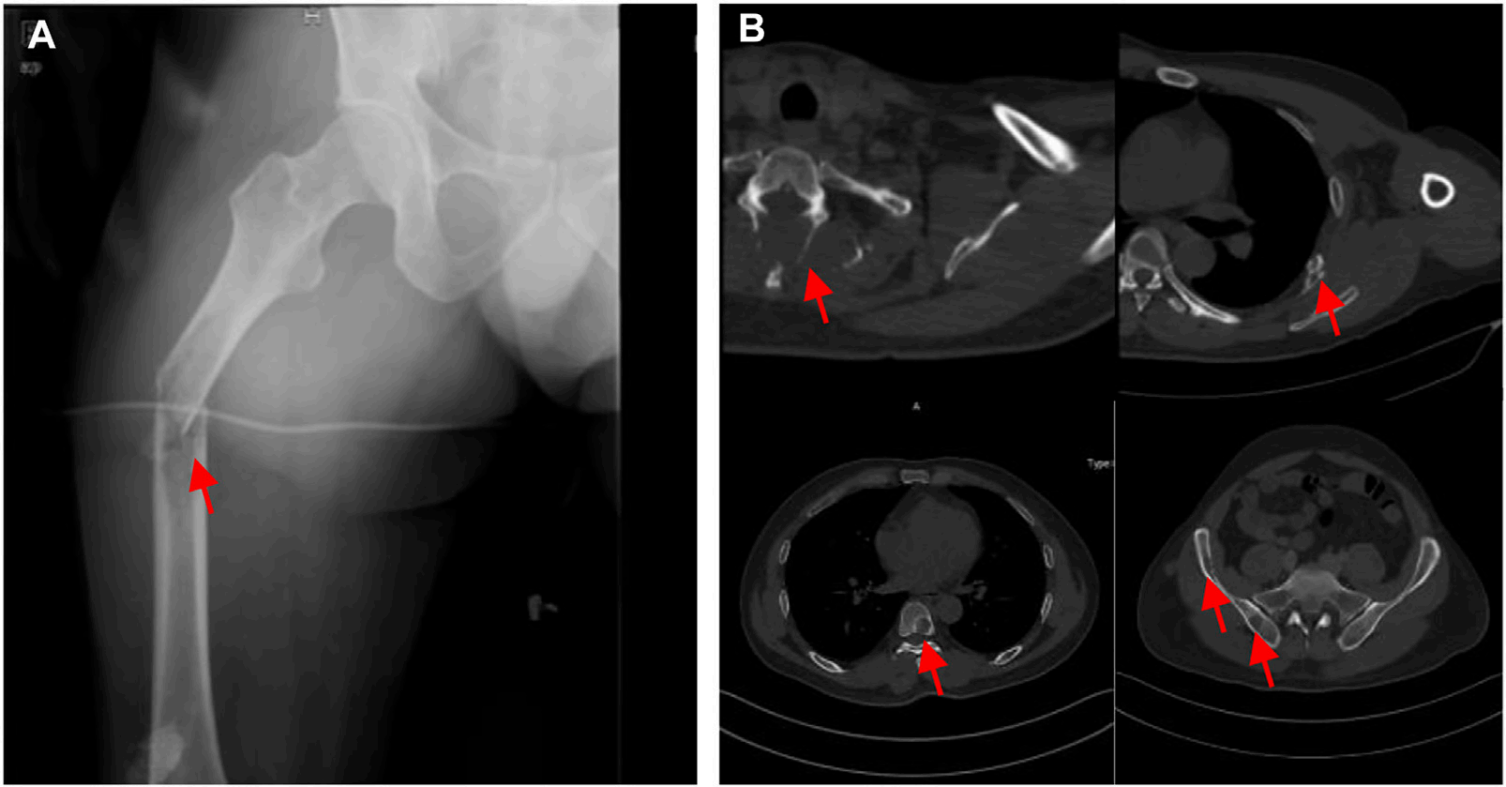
(A) Pathological fracture of the right femur. (B) Metastases at the ribs, vertebral and ilium on CT.
